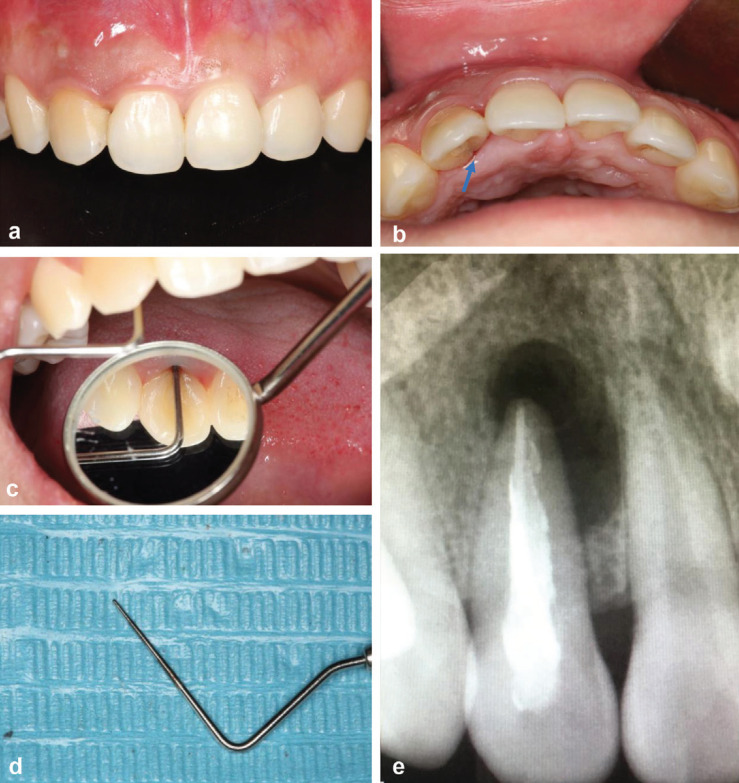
(a) The maxillary right lateral incisor. A preoperative clinical photograph shows a draining sinus tract on the labial gingiva. (b, c, d) A palate-gingival groove was detected on the maxillary right lateral incisor, accompanied by a 15-mm periodontal pocket in the mid palatal region. (e) the X-ray radiograph shows the root canal treated image and oval shadow.
